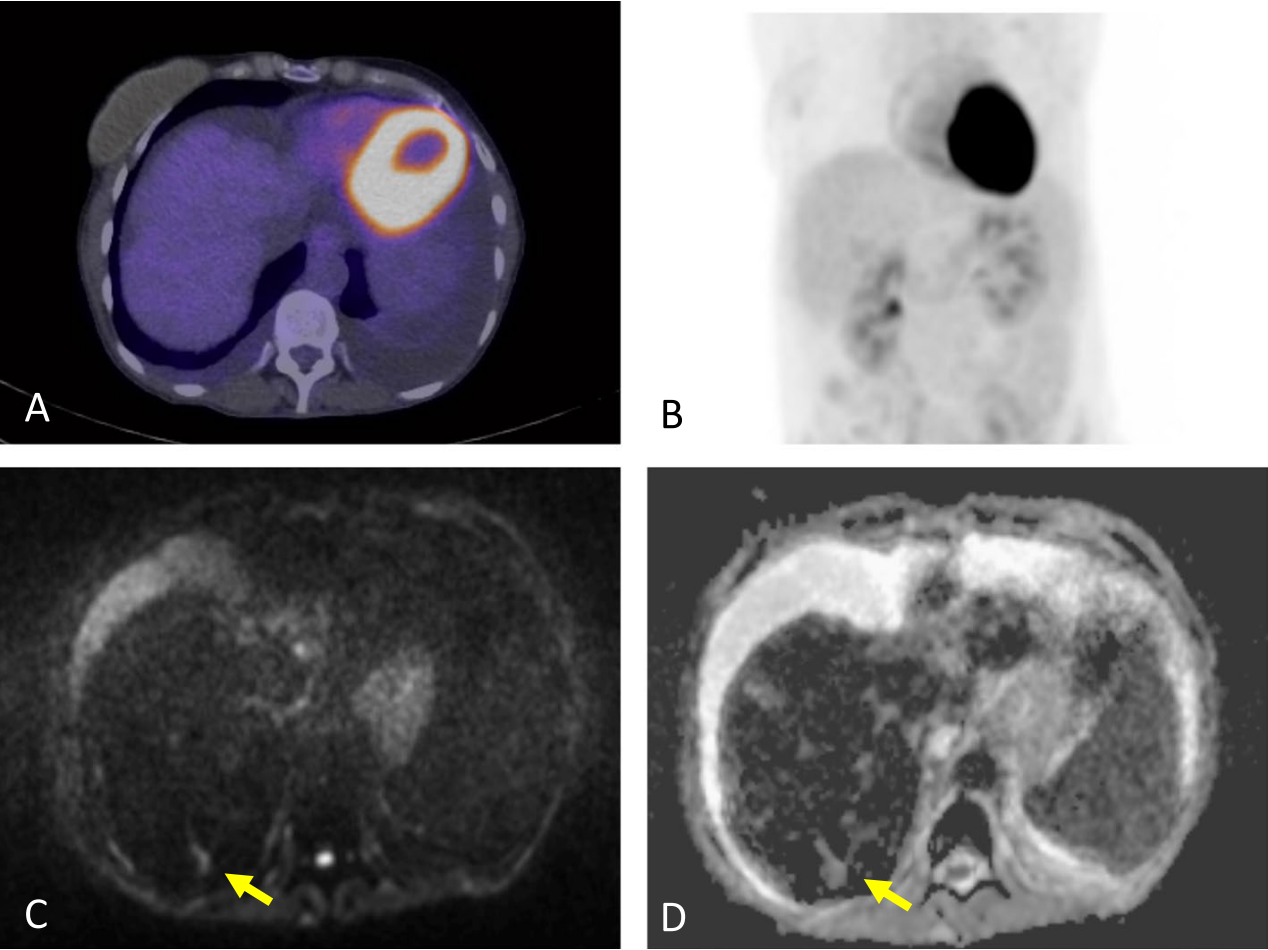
Additional sites of disease on WBMRI compared to FDG/PET-CT. 56-year-old female with ER- PR- positive HER-2 negative infiltrating ductal carcinoma with bone and liver metastases. Worsening liver function tests with decreasing haemoglobin and platelet count were noted whilst on Exemestane. Fused PET-CT images (A) and FDG/PET-CT MIP (B) showed no significant pathological activity in the liver. There is pseudocirrhosis, ascites and pleural effusions. High b-value (b900) image (C) and corresponding ADC map (D) of a WBMRI study undertaken four weeks later demonstrated multiple liver metastases measuring up to 20 mm in the posterior right hepatic lobe (yellow arrow) not appreciated on the FDG/PET-CT study
